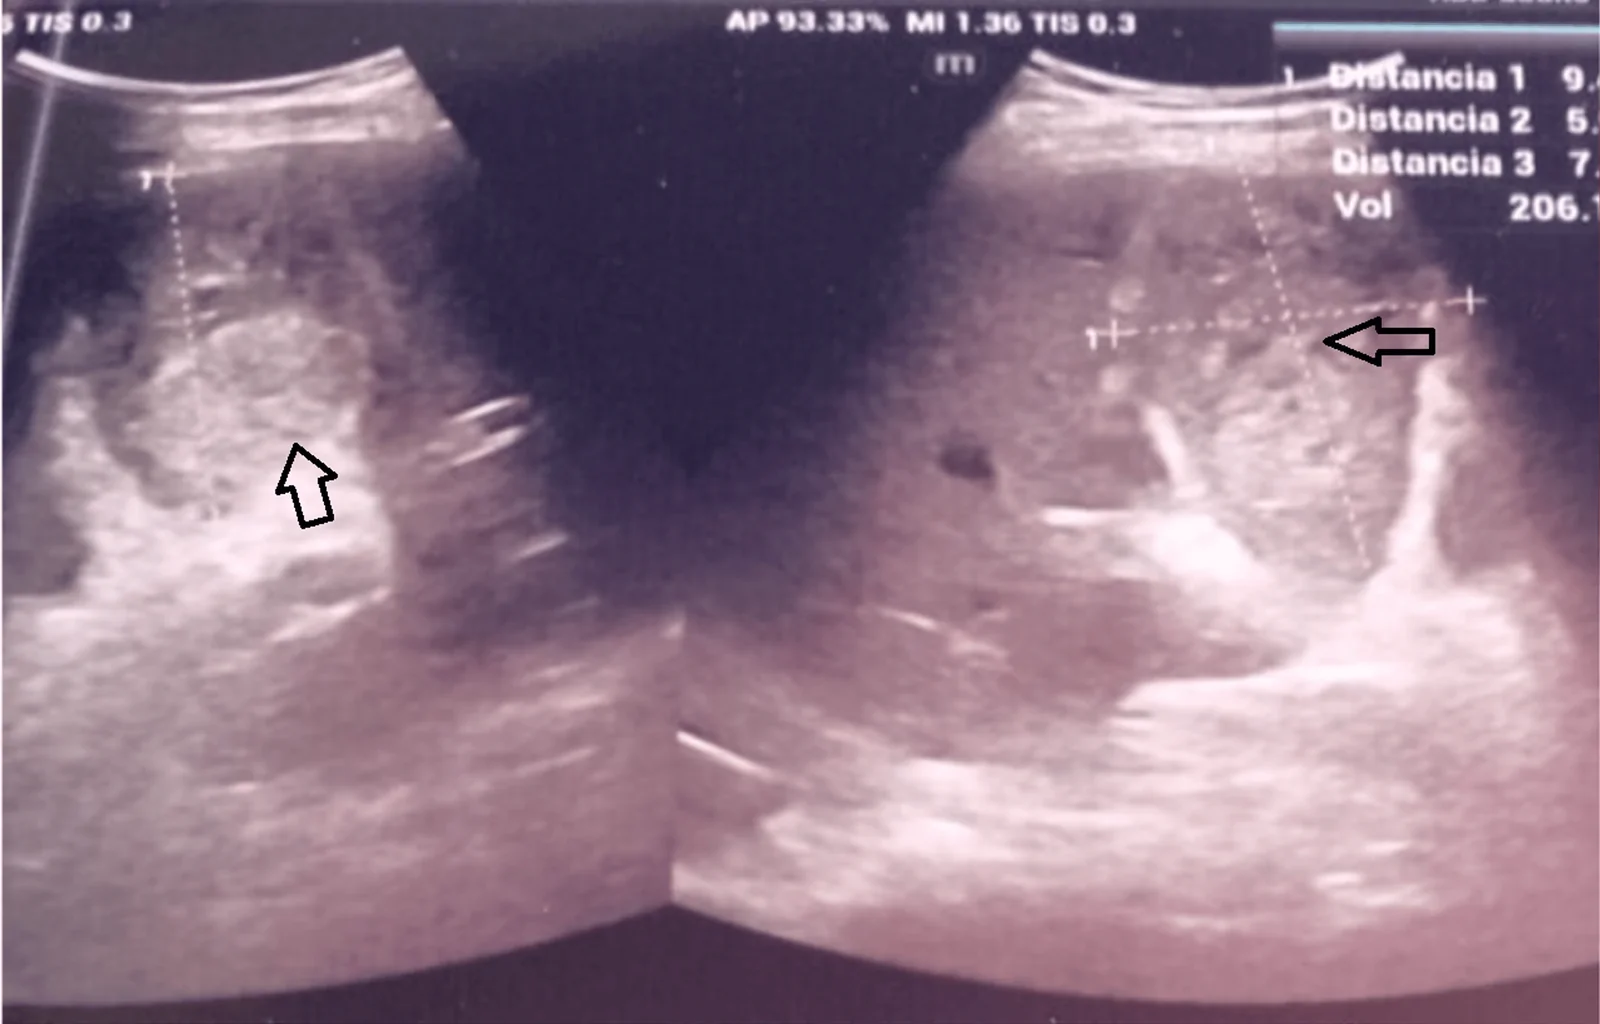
Abdominal ultrasound shows a distended GB with echogenic intraluminal content without acoustic shadowing (arrowheads), consistent with hemorrhagic material. Free intraperitoneal fluid is visible in the hepatorenal space, consistent with hemoperitoneum. The two images are the same ultrasound, with two sides or points of view, printed in the same sheet/film. GB: gallbladder
